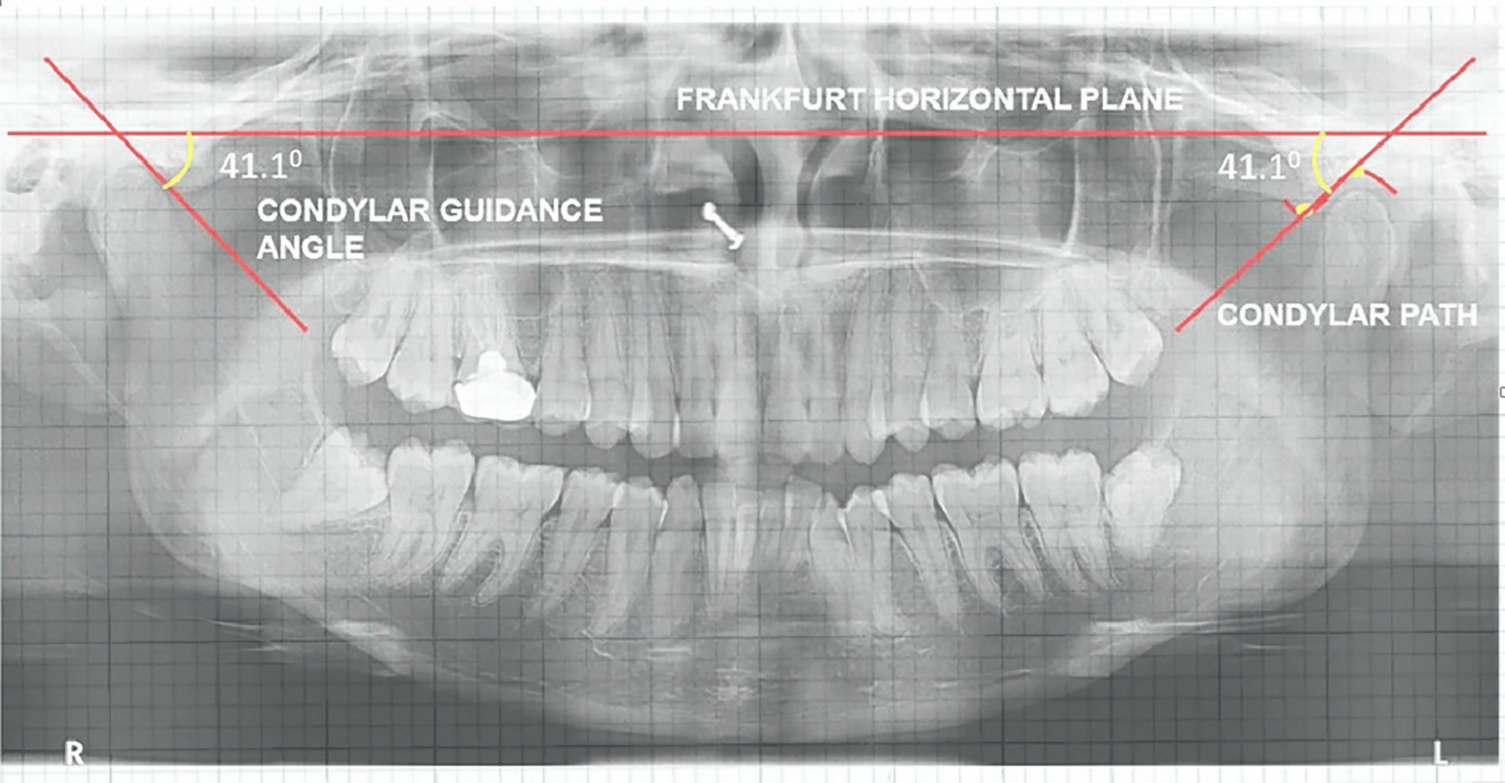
Measurement of CG angle on digitized OPG CG, condylar guidance; OPG, orthopantomogram
